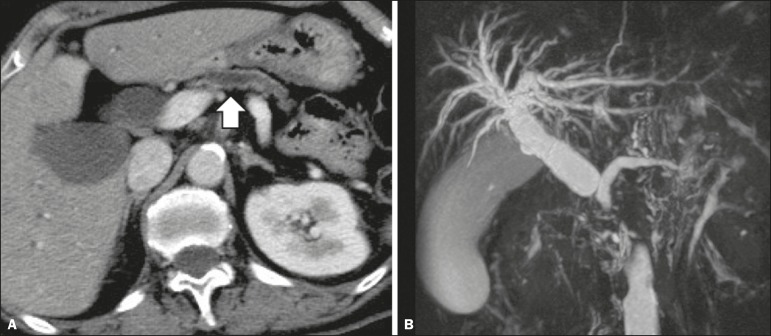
Contrast-enhanced CT (A) and magnetic resonance
